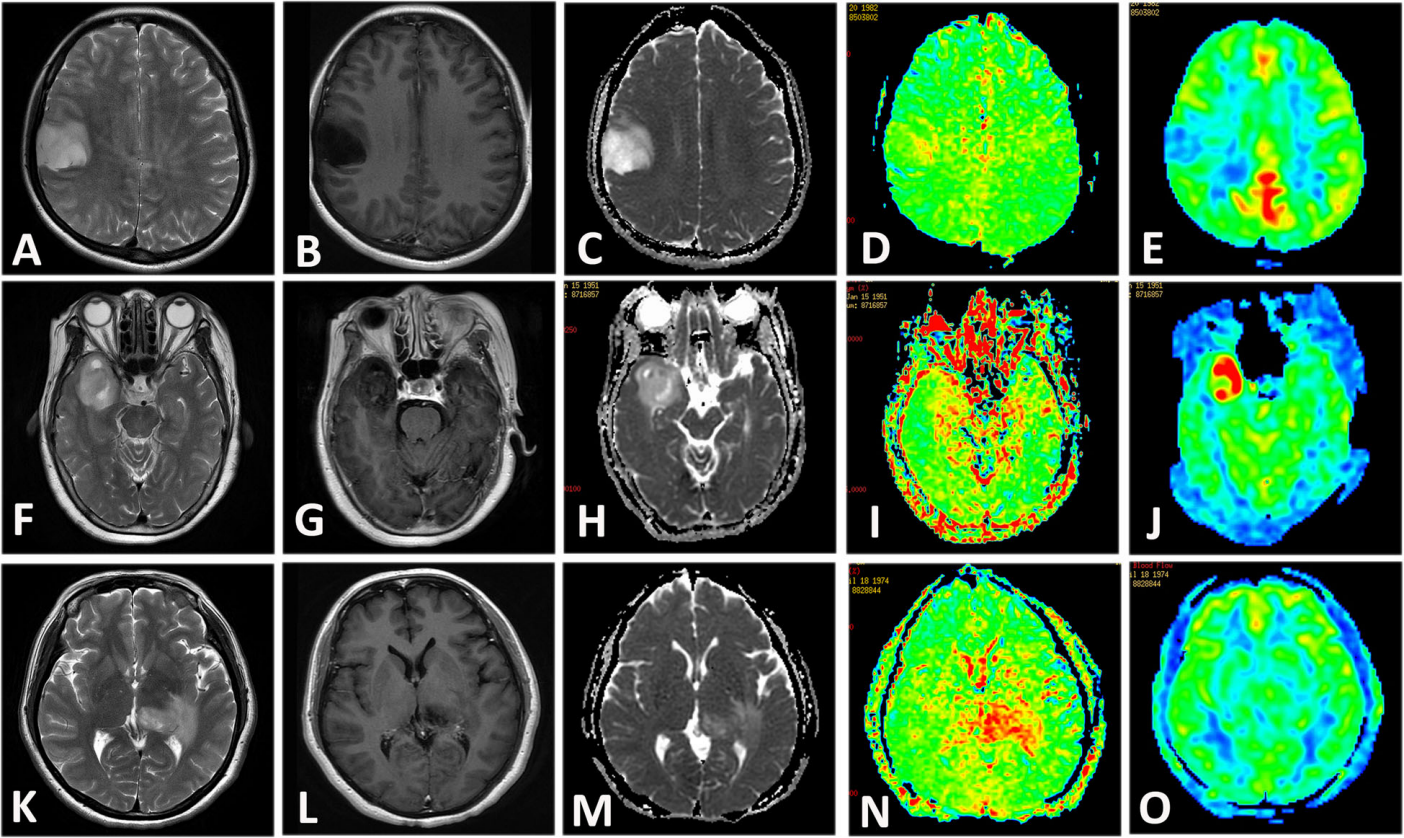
Upper row: A 35-year-old female with diffuse astrocytoma with mutant IDH1 in the right frontal lobe. A transverse T2-weighted image shows a homogeneous hyperintensity area in the right frontal lobe (a). A contrast enhanced transverse T1-weighted image shows no enhancement in the tumor (b). ADC signal was elevated (c). The APT-weighted image shows a mild increase signal in the tumor compared with normal brain tissue (d). The CBF map showed low CBF in the tumor (e). Middle row: A 66-year-old female patient with diffuse astrocytoma with mutant IDH1 showed a heterogeneously hyperintense area in the right temporal lobe in transverse T2-weighted image (f). A contrast enhanced transverse T1-weighted image shows a miner enhancement in the tumor (g). ADC signal was slightly elevated (h). The APT-weighted image shows mild increase signal in the tumor (i). The CBF map showed high CBF in the tumor (j). Lower row: A 43-year-old female with a glioblastoma with wide-type IDH1 in the left thalami and temporal lobe. A transverse T2-weighted image shows a heterogeneously hyperintense area in the left temporal lobe (k). No obvious local enhancement was observed in postcontrast T1-weighted image (l). ADC signal was not uniformly reduced (m). APT-weighted image shows high signal in the tumor compared with normal brain tissue (n). The CBF map showed no high signal in the tumor (o)
